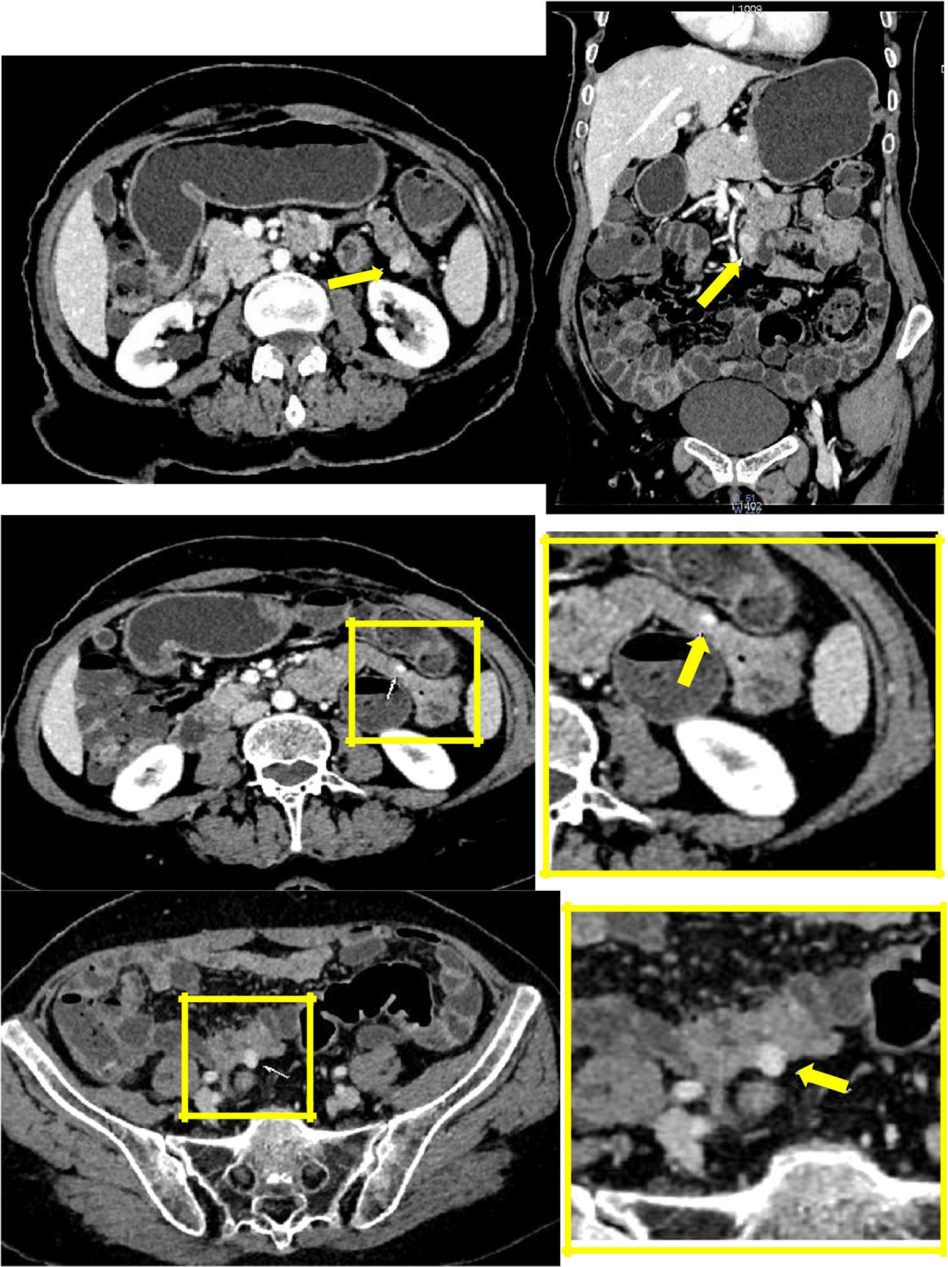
A 57-year-old female with systemic NF1. Patient presented vague abdominal pain. Dual-phase contrast-enhanced CT scan reveals multiples intestinal nodules highly enhanced by contrast (arrows)
